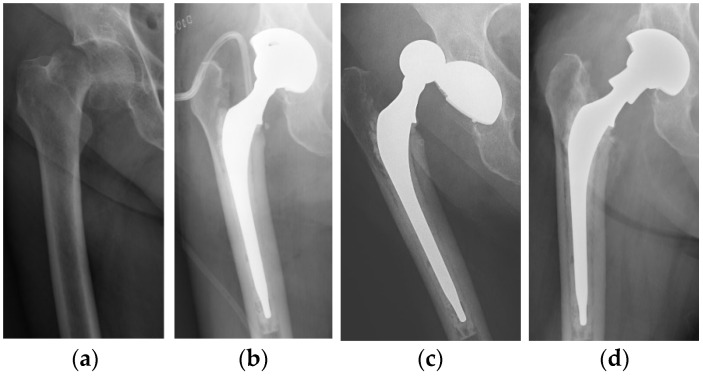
(a) Displaced intracapsular fracture (Garden-type IV) of the right femoral neck in a 79-year-old woman. (b) Postoperative radiograph after surgical treatment with a conventional THA. (c) Prosthesis dislocation 3 weeks after surgery. (d) Radiograph after implantation of a neck adaptor to increase length and lateral offset.
